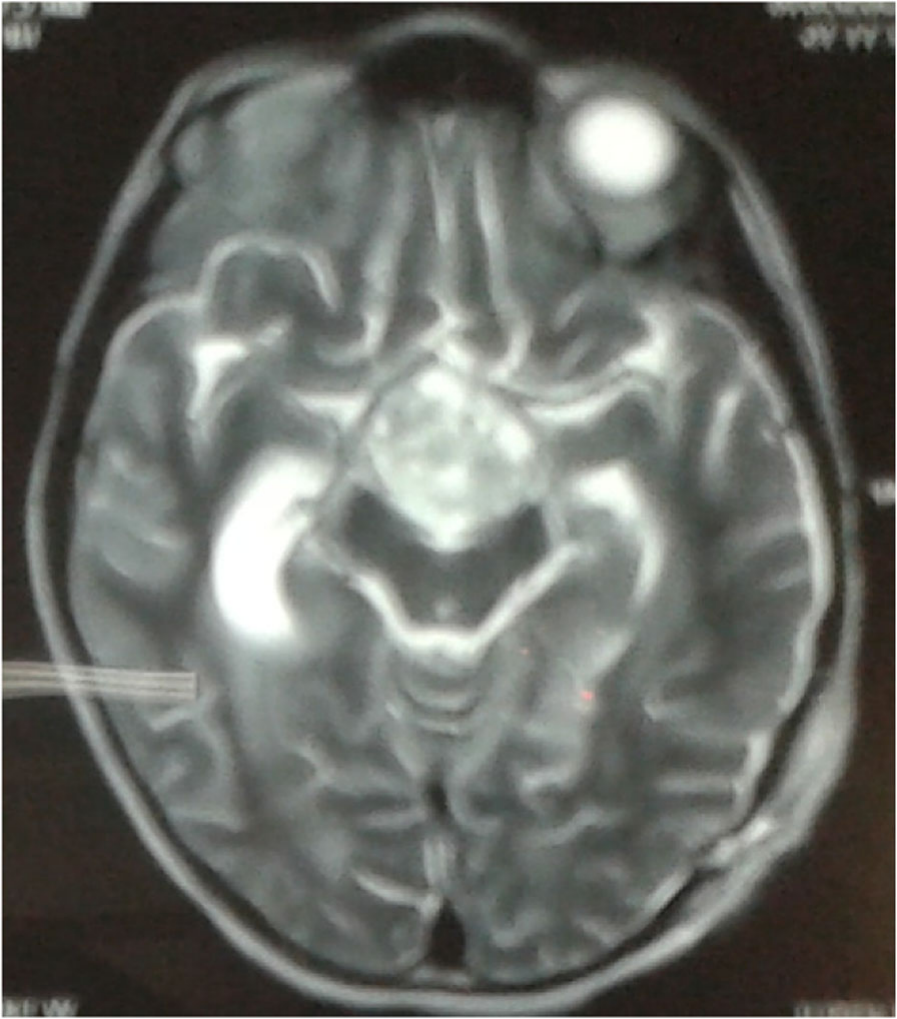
Magnetic resonance imaging T2-weighted pre-contrast axial image of the lesion
